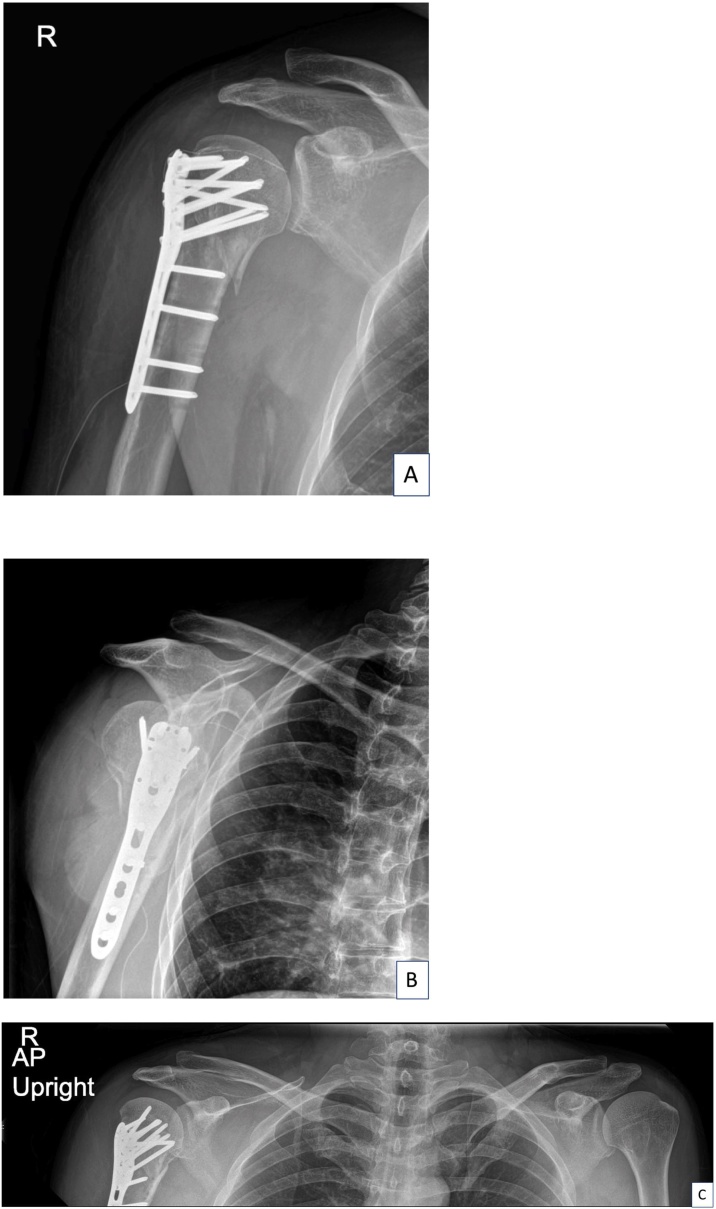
Postoperative radiographic imaging: (A) anteroposterior view of right shoulder, (B) transcapular view of right shoulder, and (C) anteroposterior view of both clavicles.
